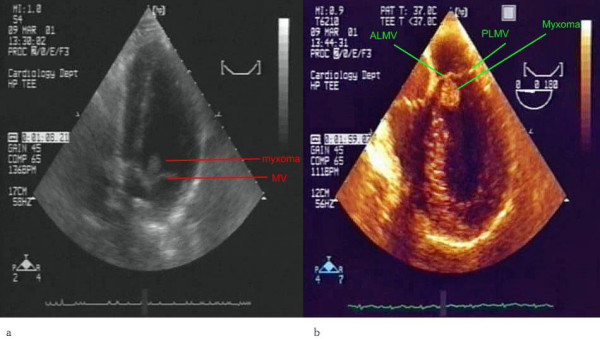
Myxoma originating from the anterior mitral valve leaflet. a. Transthoracic echocardiographic study, apical 2 chamber view. Myxoma seen attached at the ventricular surface of the anterior mitral valve leaflet (dimensions 1,99 × 1,1 cm). b. Transoesophageal echocardiographic study, 4 chamber view. Left ventricular myxoma arizing from a thin stalk attached at the ventricular surface of the anterior mitral valve leaflet. ALMV refers to anterior leaflet of mitral valve and PLMV refers to posterior leaflet.
